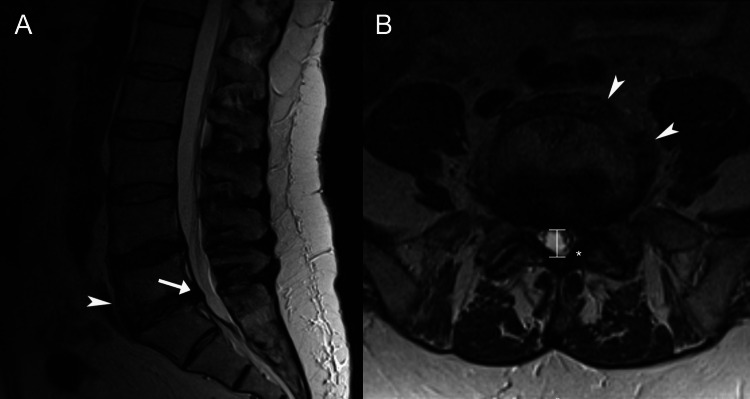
Lumbar MRI. The T2-weighted mid-sagittal view (A) demonstrates disc displacement at L5/S1 which measures 6 mm in anteroposterior diameter (arrow). The axial view of L5/S1 (B) better demonstrates a central disc bulge at this level, causing moderate spinal canal stenosis and moderate left and mild right neural foraminal narrowing. The anteroposterior diameter of the spinal canal (“I” marker) is narrowed to nine millimeters. In addition, the ligamentum flavum (*) is slightly thickened, measuring 5 mm in thickness on the left side, and contributes to the canal stenosis. As the axial view is not perfectly parallel with the disc plane, anterior osteophytes are also well visualized in this view (arrowheads), which measure a maximum 10 mm from the vertebral body. The disc bulge at L4/5 is smaller and does not cause any significant foraminal narrowing (not indicated).
